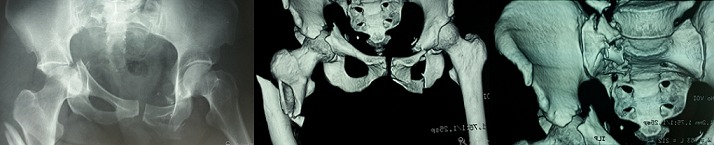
(A) radiographie du bassin face; (B) TDM du bassin avec reconstruction 3D; (C) TDM bassin montrant la fracture du cotyle, du sacrum et du rachis
